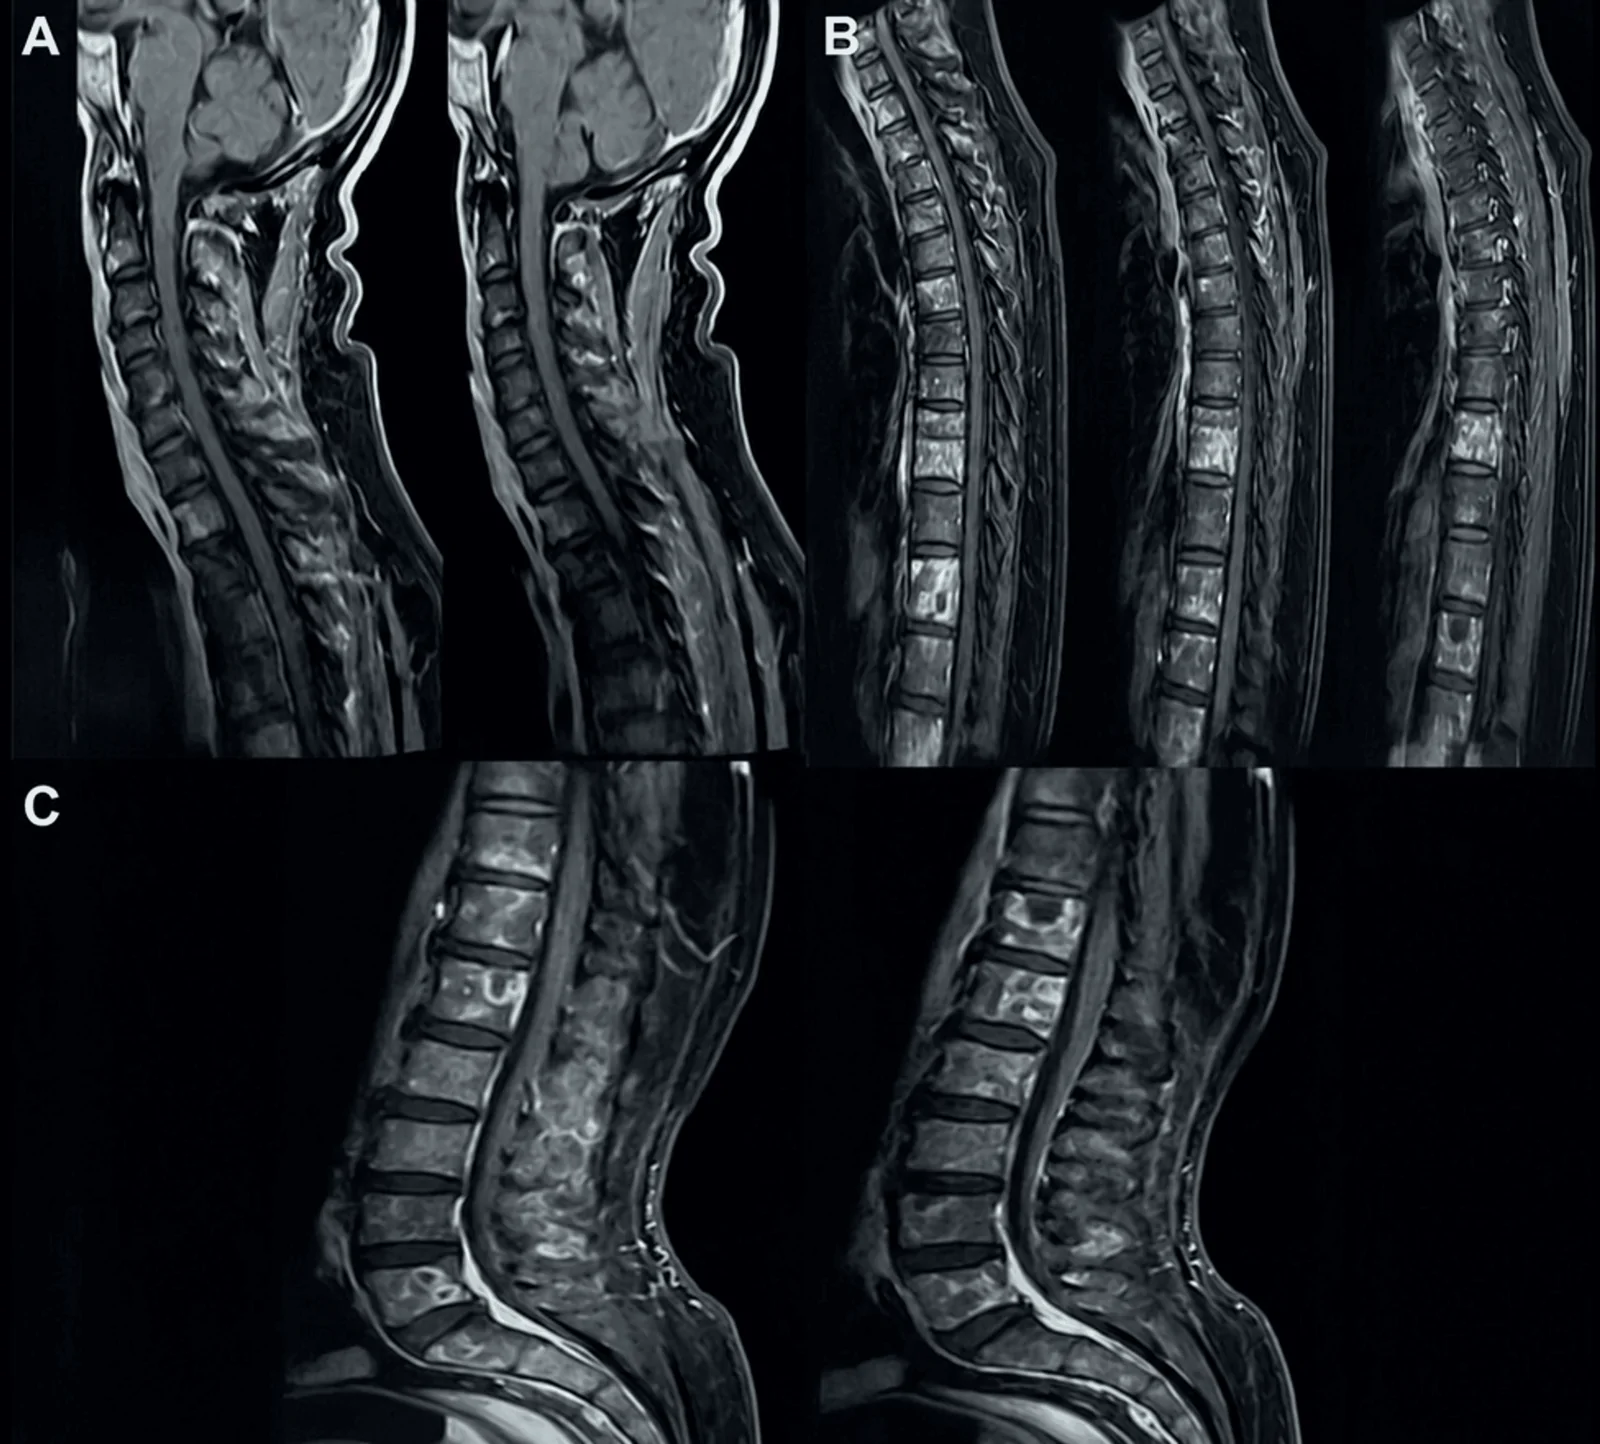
Sagittal STIR MRI of the cervical (A), thoracic (B), and lumbosacral (C) spine demonstrating diffuse hyperintense marrow lesions involving multiple vertebral bodies, consistent with extensive osseous metastatic disease and diffuse bone marrow infiltration
